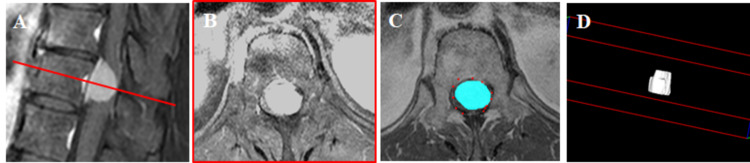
Tumor location and measurement of tumor volume. (A–D) Meningioma (spinal canal type). (A) T2-weighted MR images sagittal plane. (B) T2-weighted MR images axial plane. (C) Contrast-enhanced MR imaging axial plane. (D) The region of the tumor was isolated and a three-dimensional model was created. MR, magnetic resonance.
